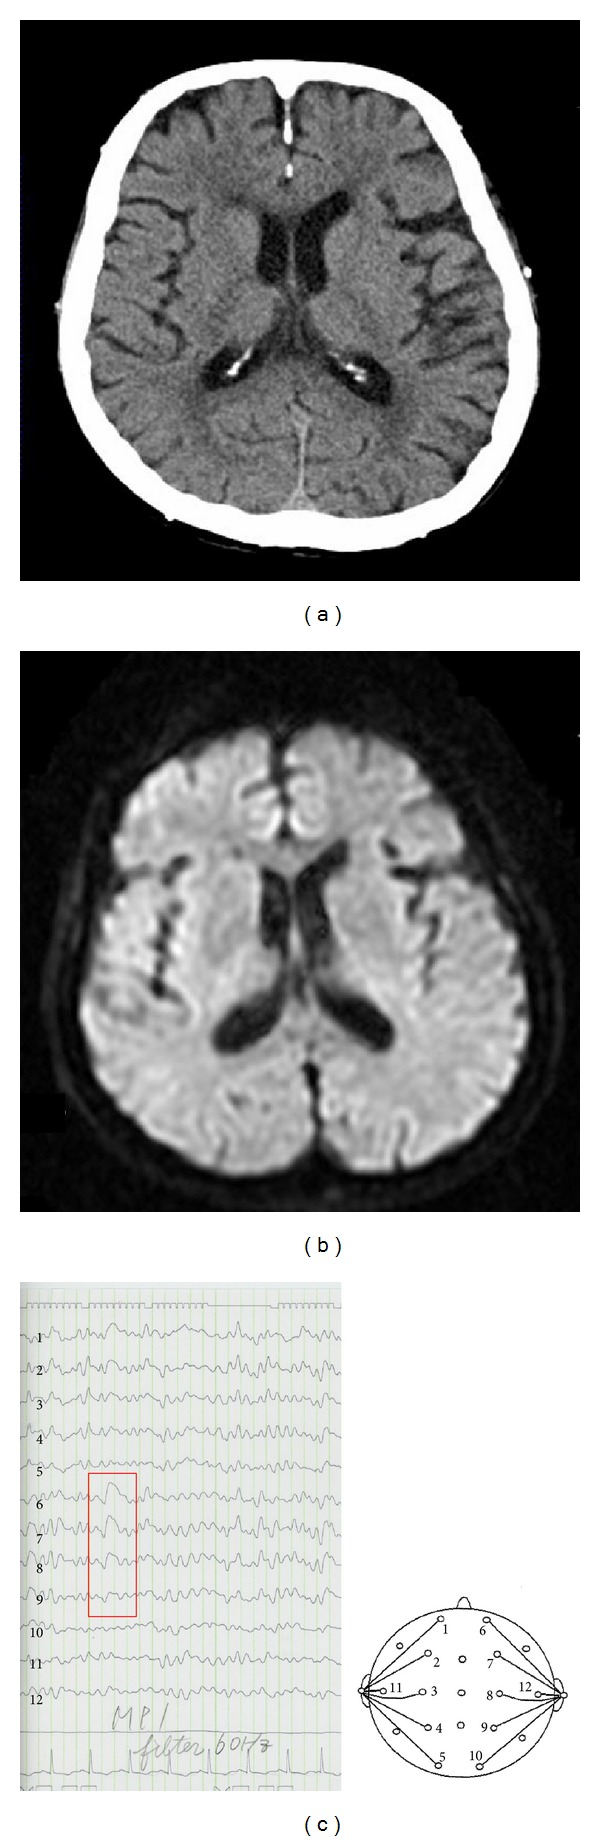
Case 1. Brain computed tomography (CT) and magnetic resonance imaging (MRI) on admission. Brain CT showed no acute cerebral bleeding (a). Brain MRI showed no acute cerebral infarction (b). Electroencephalography after admission showed repetition of intermittent high-amplitude irregular slow waves in the right frontal lobe (c). *Lead placement: 1, left frontal pole (Fp1); 2, left frontal (F3); 3, left central (C3); 4, left parietal (P3); 5, left occipital (O1); 6, right frontal pole (Fp2); 7, right frontal (F4); 8, right central (C4); 9, right parietal (P4); 10, right occipital (O2); 11, left mid temporal (T3); 12, right mid temporal (T4).
